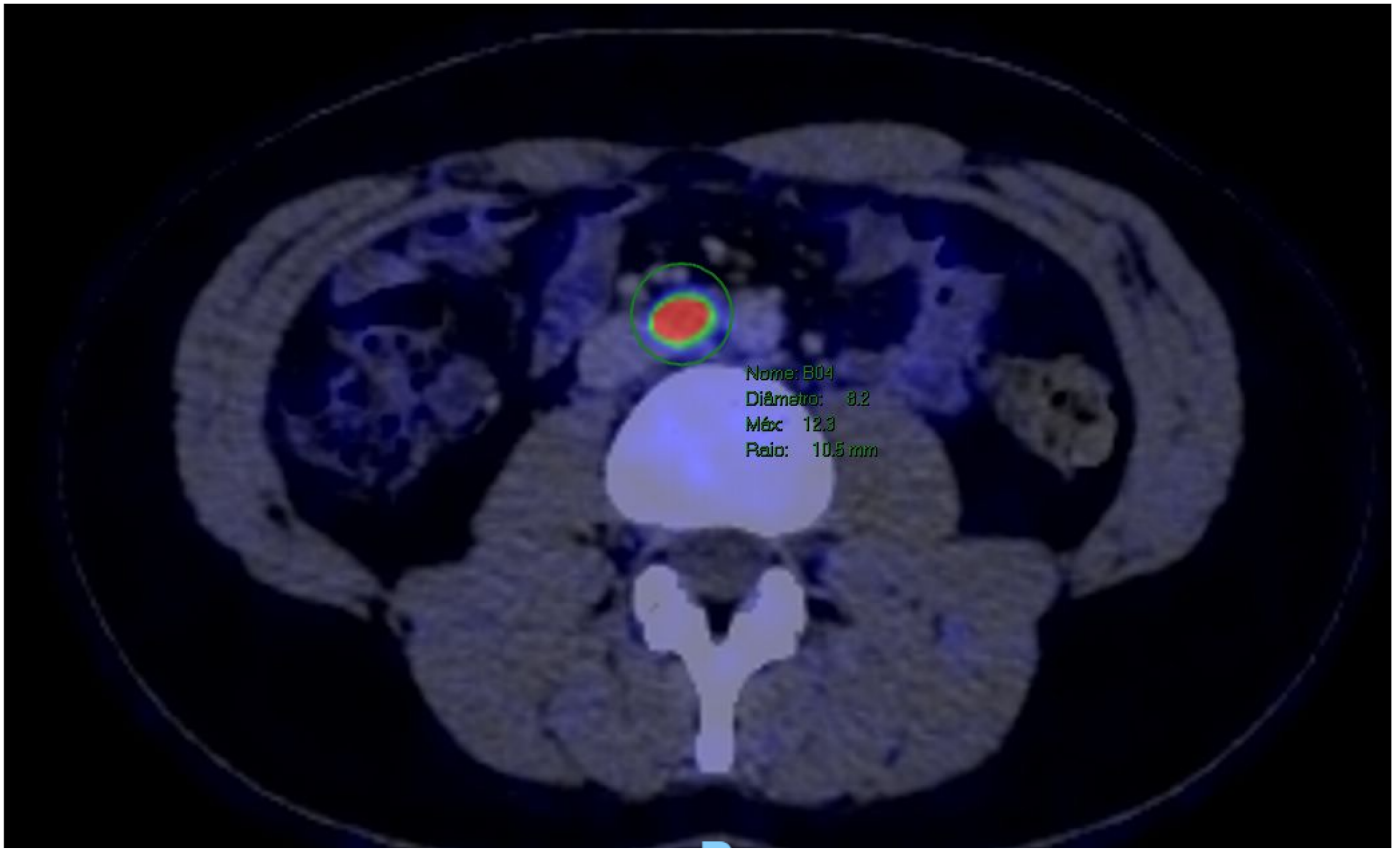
PET-CT showed a lymph node with marked hypermetabolism in the interaortocaval region.
